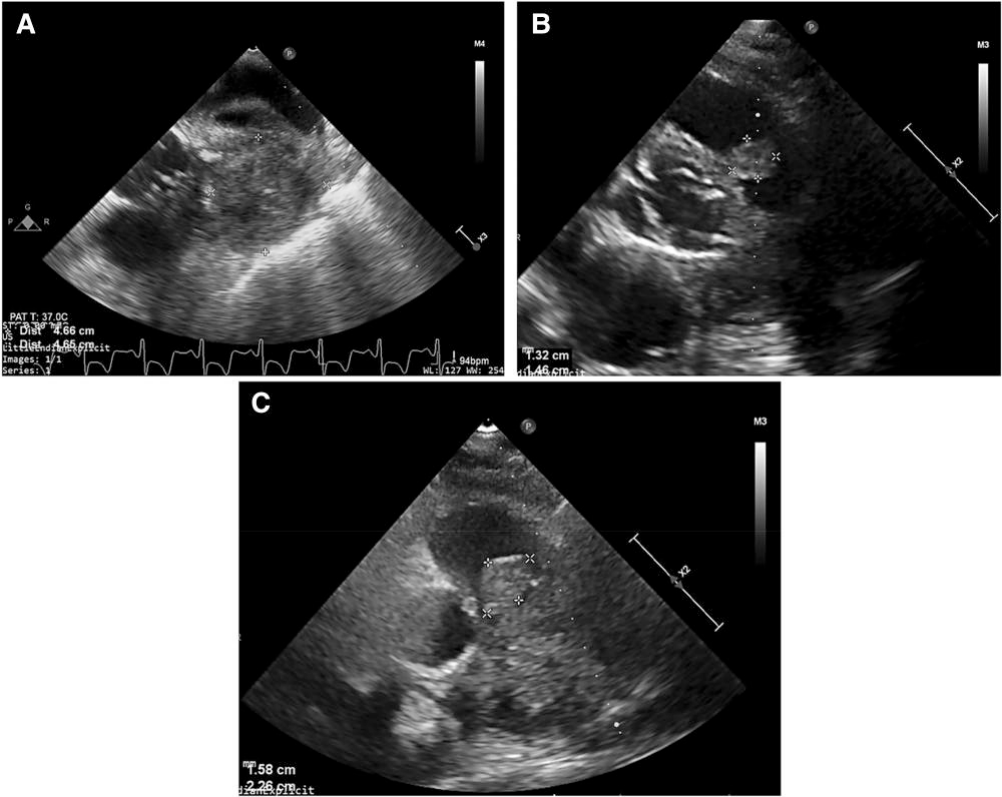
(*A*) Post-intervention transoesophageal echocardiography (modified midoesophageal short-axis view) reveals the partially thrombosed giant PSA (right lateral side of the image), while the mitral valve is free of thrombi or vegetations (left lateral side of the image). (*B*) Parasternal short-axis view reveals a vegetation attached to one of the cusps of the pulmonary valve at re-admission for septic status. (*C*) Parasternal short-axis view at the pulmonary valve level visualizing the vegetation with increasing dimensions after re-admission for septic status.
